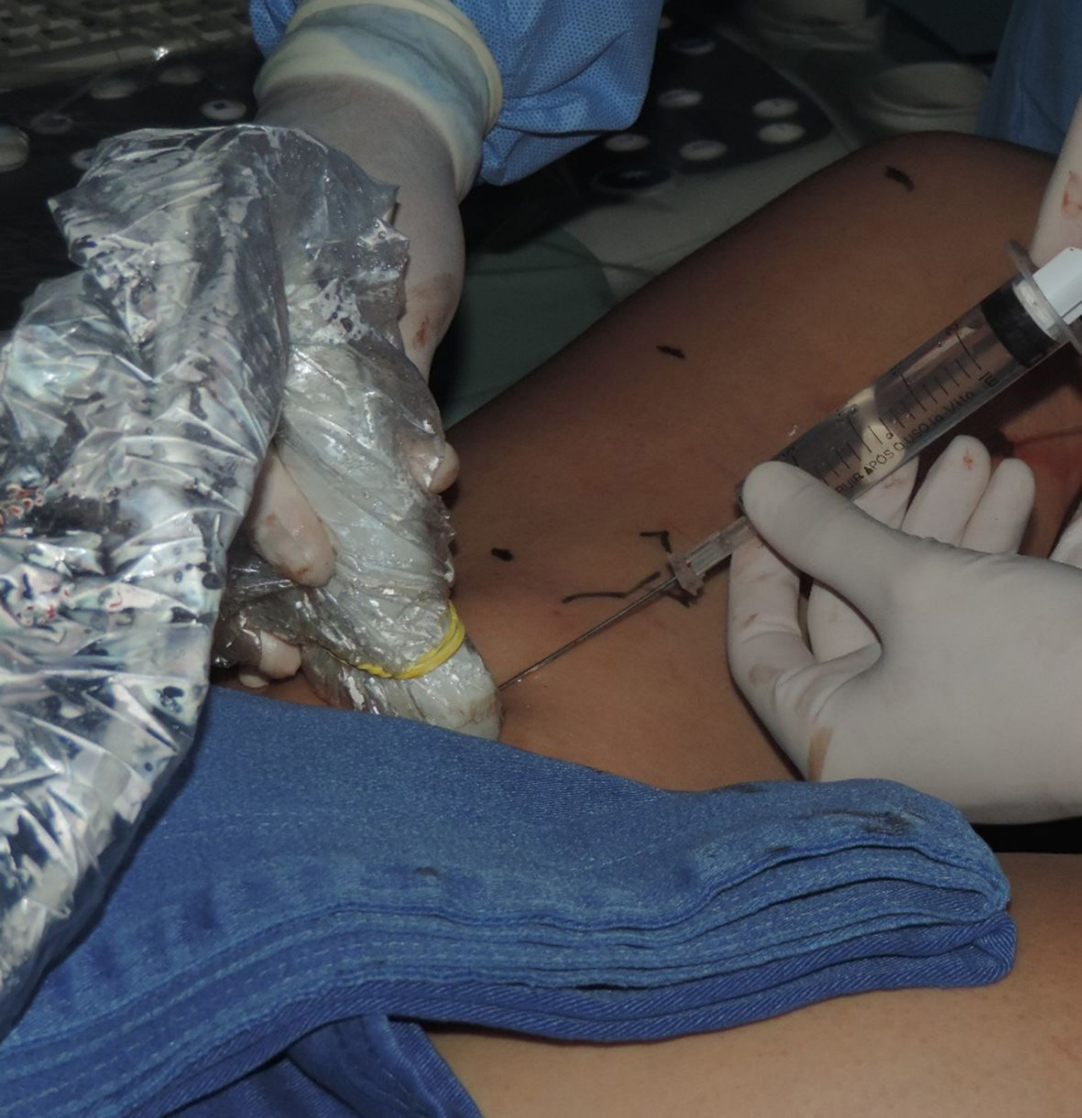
Initiating tumescent anesthesia induction with ultrasound control.
